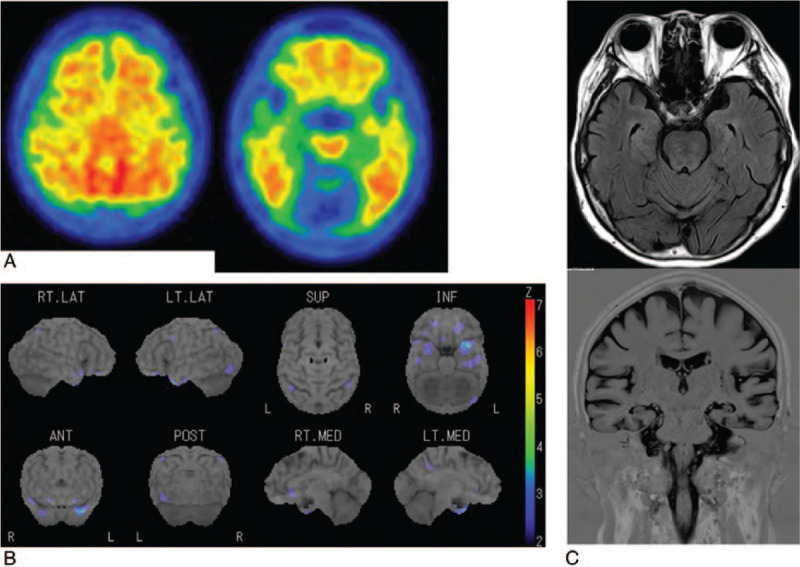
A 78-year-old woman with amnestic MCI (Case 16). a. ^11^C-PiB PET shows positive amyloid burden with, a mean cortical standardized uptake value ratio (SUVR) of 1.90. b. ^123^I-IMP SPECT and color-coding representing the statistical significance of decrease in regional cerebral blood flow as compared to normal does not show hypoperfusion in any parietotemporal regions, posterior cingulate gyrus and precuneus. c. Axial and coronal MRI shows no hippocampal atrophy, with a Z-score of 1.88.
